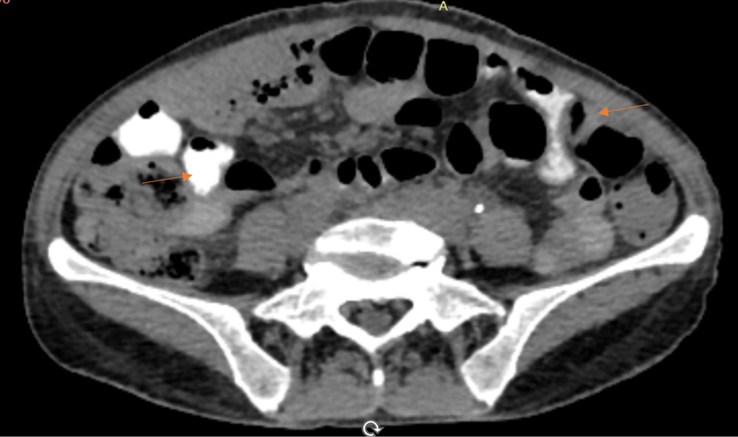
Axial section of contrast-enhanced computed tomography (CECT) showing filling of jejunal loops with contrast via the recto-jejunal fistulas (Orange thin arrows).
